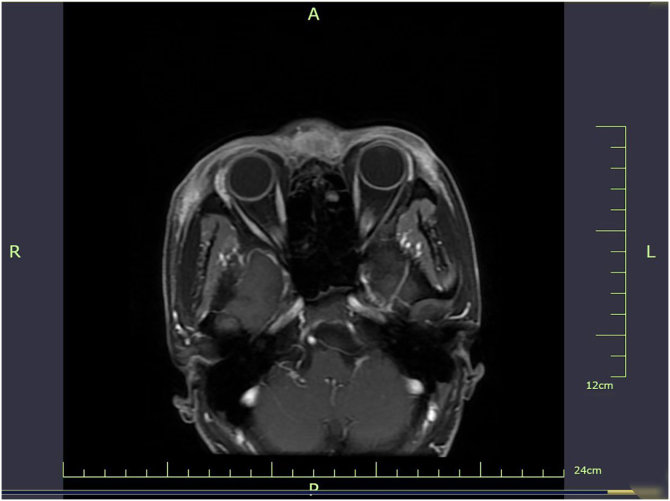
Enhanced MRI (pretreatment): The subcutaneous soft tissues of the frontotemporal region and periorbital area demonstrate thickening, with a maximum thickness of approximately 1.5 cm. Patchy foci of isointense T1 and slightly hyperintense T2 signals are identified, showing ill-defined margins and heterogeneous signal intensity. Post-contrast imaging reveals homogeneous enhancement of these lesions; on T2WI sequences, interspersed areas of hyperintense T2 signals are noted within the lesions, which also exhibit homogeneous enhancement following contrast administration.
